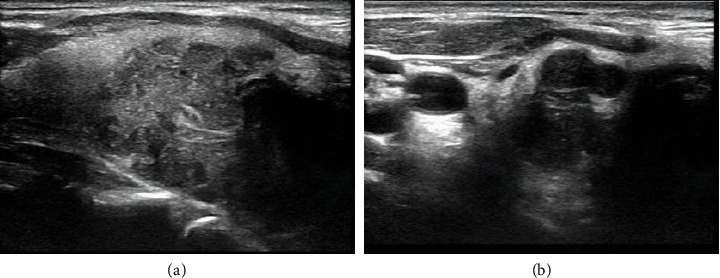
Preoperative ultrasound images of neck. (a) The image showed a large hypoechoic nodule on the right lobe of thyroid. (b) The image showed a lot of multiple circular and hypoechoic abnormal cervical lymph nodes.
